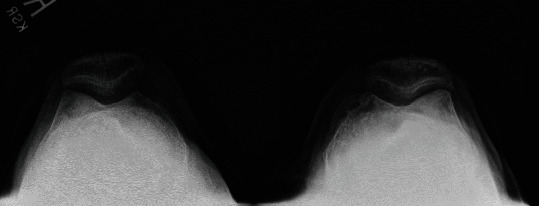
Preop merchant knee X-ray.
